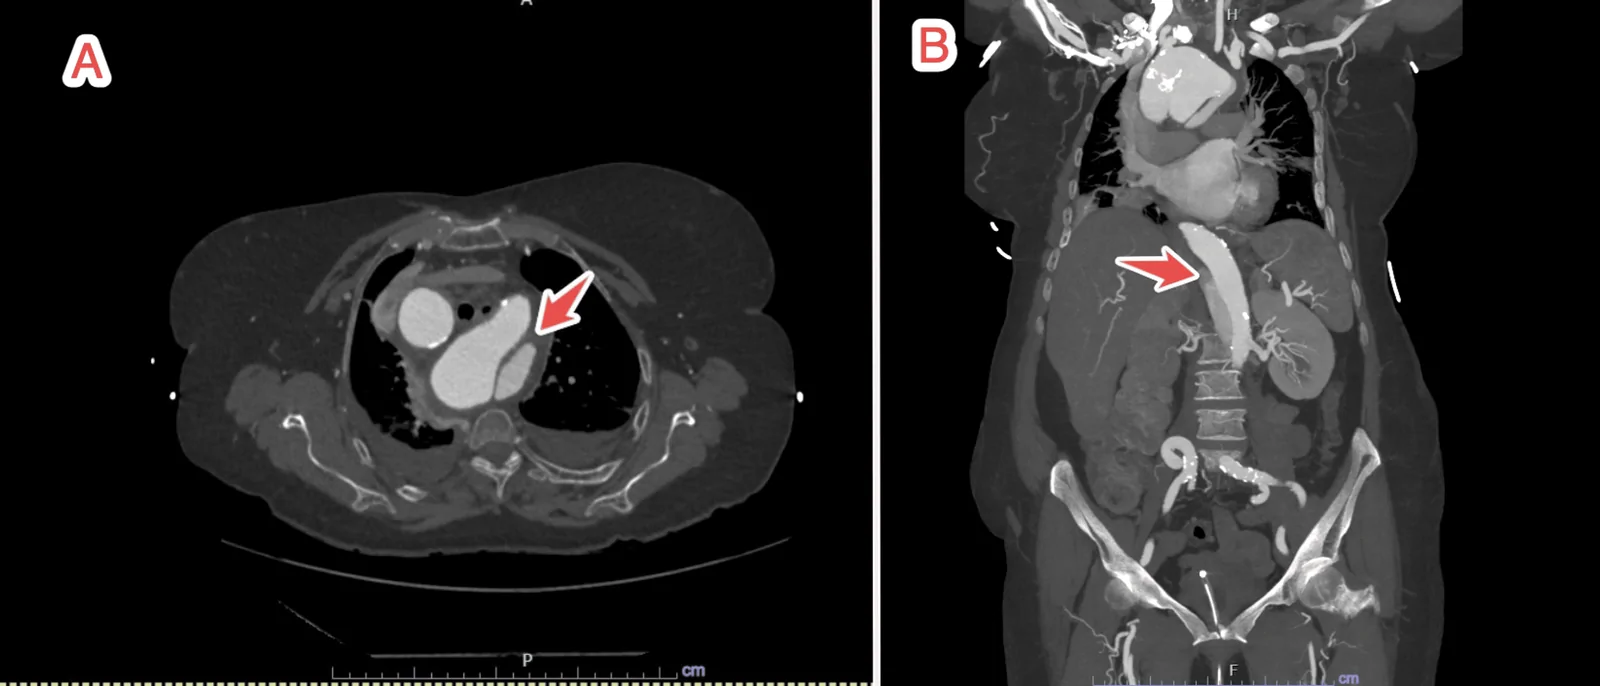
Preoperative computed tomography angiography showing acute Stanford type B aortic dissection. (A) Axial contrast-enhanced computed tomography image showing the dissected descending thoracic aorta with the intimal flap separating the true lumen from the patent false lumen. (B) Coronal reformatted image showing longitudinal extension of the dissection through the descending thoracic and abdominal aorta. The arrows indicate the dissection flap and patent false lumen.
